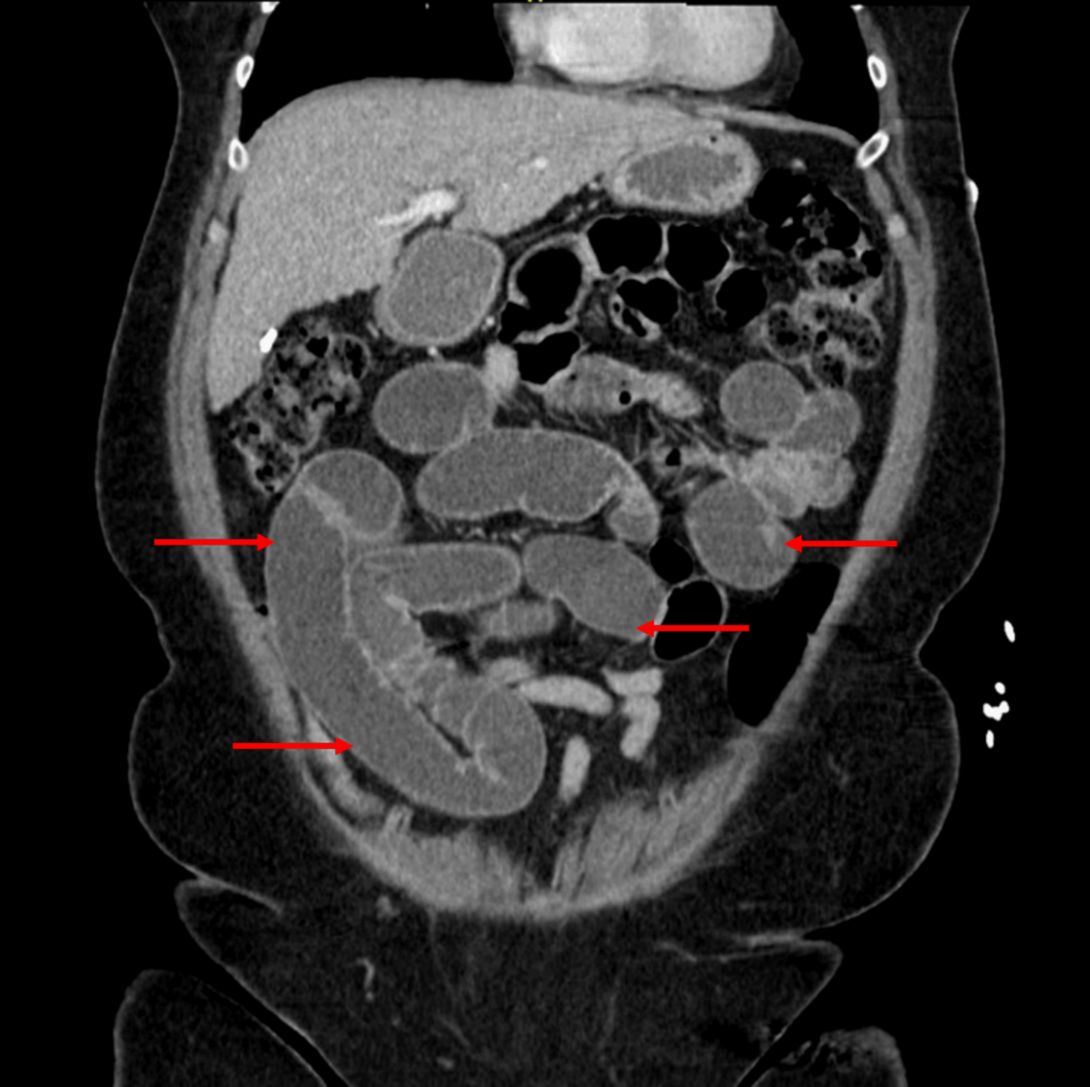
CT scan (coronal view) showing dilated loops of bowel (shown by red arrows)
